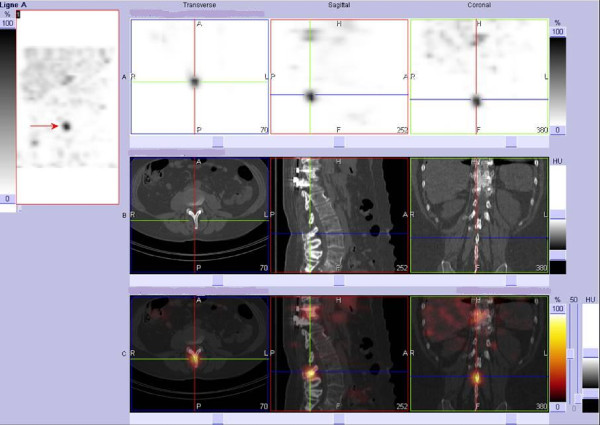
Single photon emission computer tomography (SPECT) I-131-CT demonstrating a persistent hyperfixation at the operated level and even higher fixation at the spinal process of L3.
